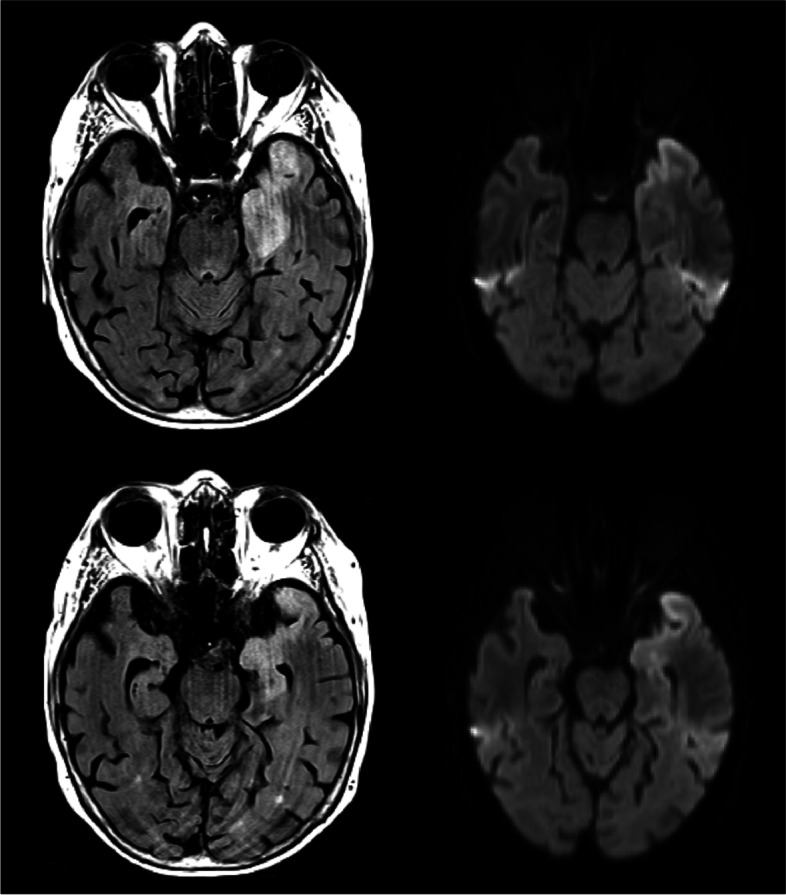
Brain MRI in a patient with encephalitis and positive HSV DNA and Caspr2 antibodies. Legend: T2-weighted MRI shows hyperintense lesion in the left temporo-mesial lobe (left) with diffusion restriction on the diffusion-weighted sequences (right)
